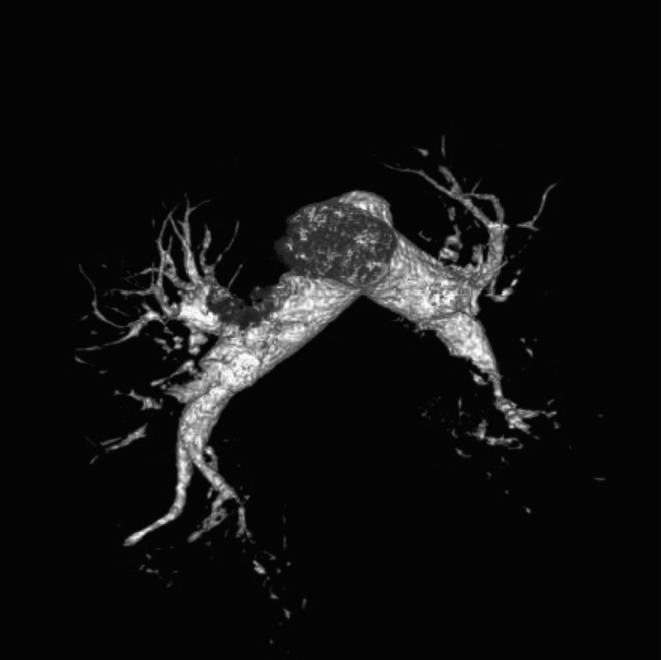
Contrast‐enhanced CT pulmonary angiography demonstrating multiple filling defects within the pulmonary arterial branches consistent with acute pulmonary emboli in the setting of severe gastrointestinal bleeding–associated hypercoagulability.
